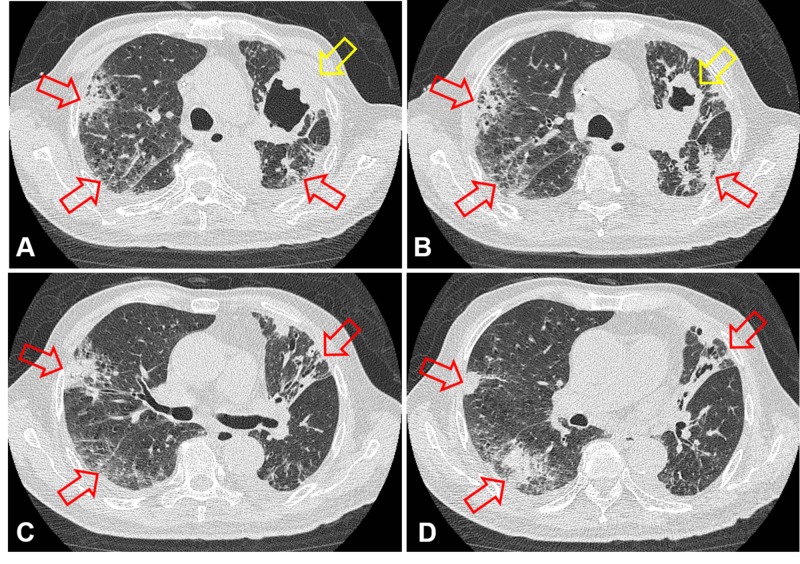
Tumor cavitation (yellow arrows) and multiple foci of pneumonitis (red arrows) on sequential axial CT scan (lung window): A to D. June 12, 2019
